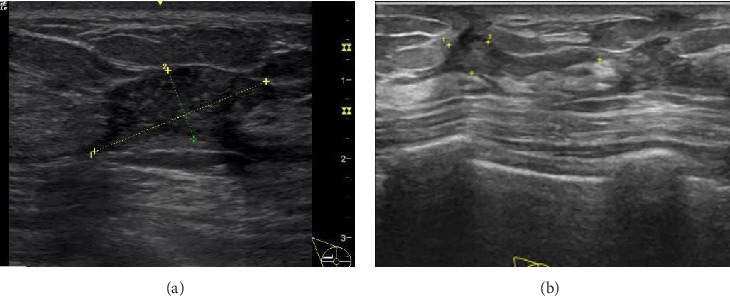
Treatment outcomes of the enrolled patients. (a) Lesion volume detection under ultrasonography for a typical case at 3 months after MWA. (b) Lesion volume detection for a typical case under ultrasonography at 5 months after MWA.
